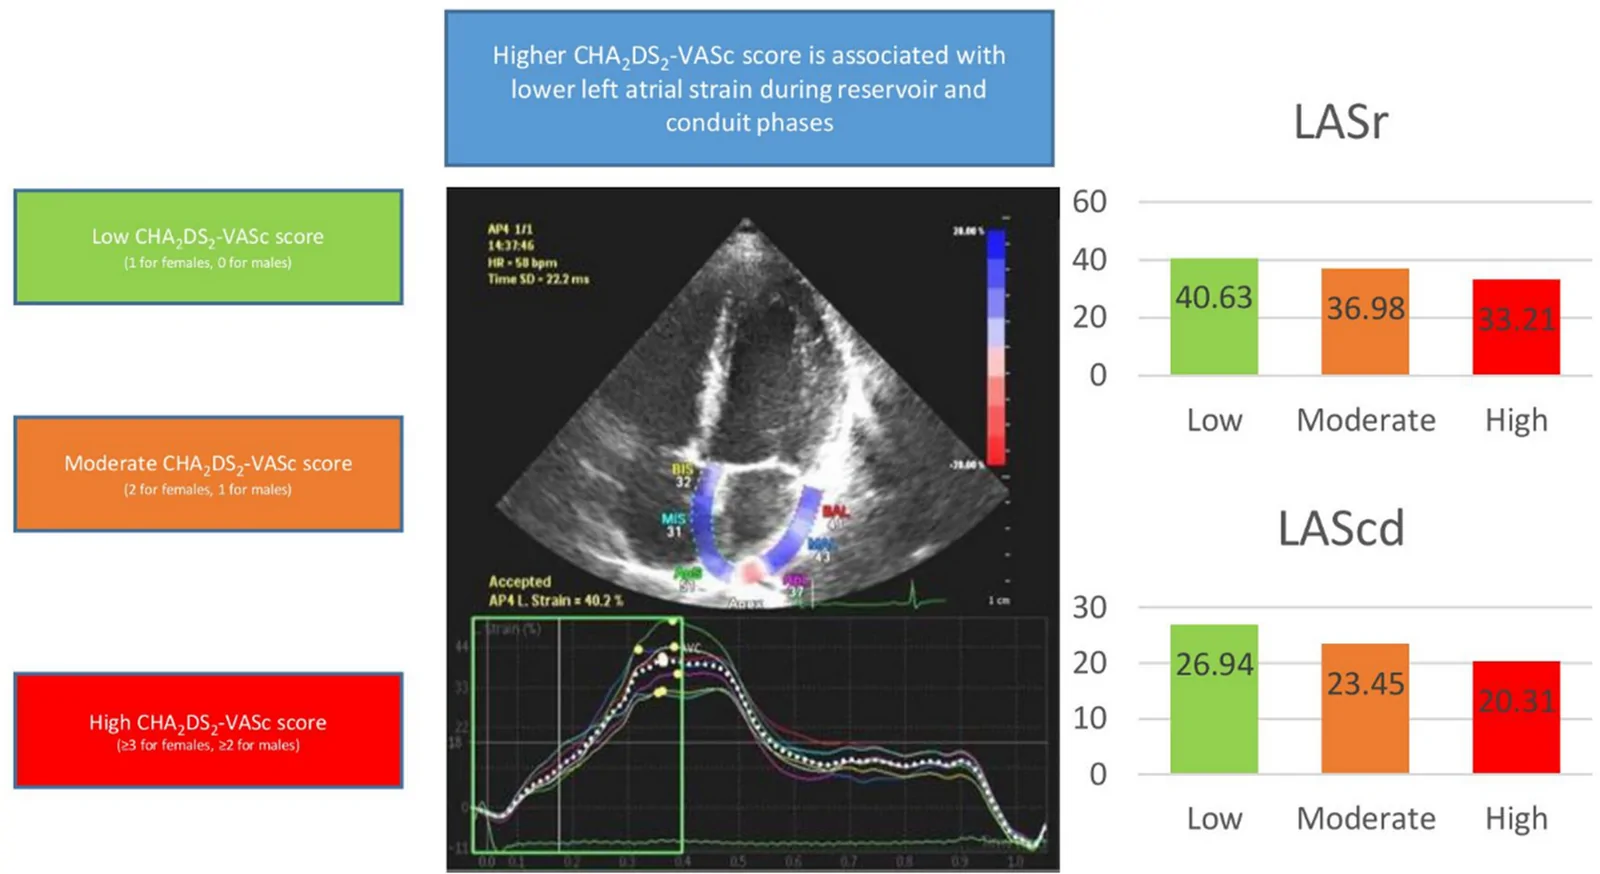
Speckle-tracking echocardiography across different CHA_2_DS_2_-VASc risk score categories shows lower left atrial strain during reservoir and conduit phases with increasing risk score. LAScd, left atrial conduit strain; LASr, left atrial reservoir strain
